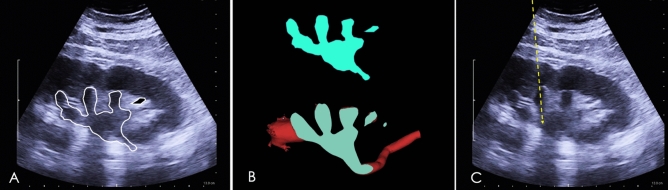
Accurate navigation of the puncture through the axis of the targeted renal calyx. (A) Ultrasonographic (US) observation of the section profile (white contour) of the renal collecting system. (B) US profile of the renal collecting system (superior blue contour) was highly consistent with the optimal axial section C (inferior blue contour). (C) Direction of the ultrasonic probe was now fixed, and the puncture route (yellow arrow) through the center of the targeted renal calyx section was the axis of the targeted renal calyx.
